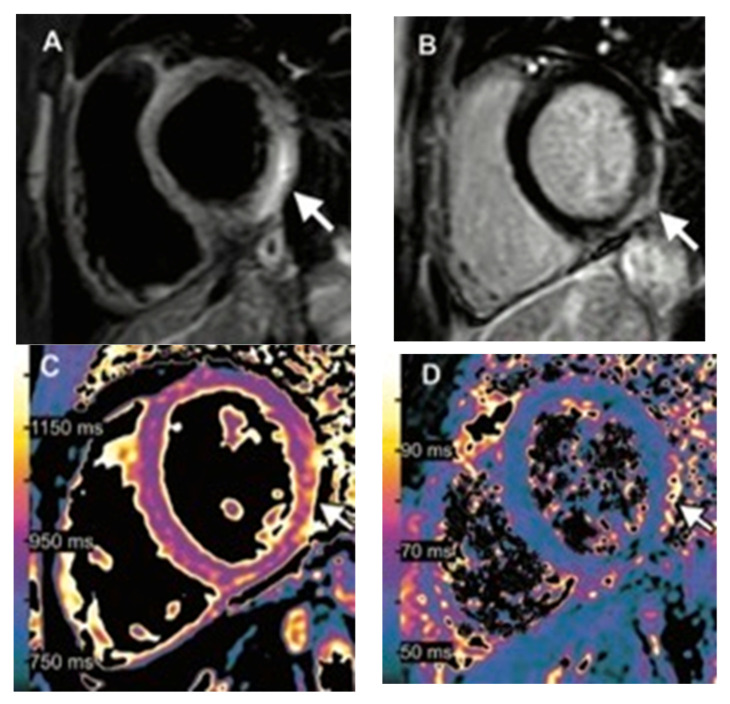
Images of a 15-year-old boy with myocarditis after COVID-19 vaccination. One day after receiving his second vaccination dose, he developed fever, myalgia, and intermittent tachycardia. (A) T2-weighted short inversion time inversion recovery MRI scans at 1.5 T in short-axis view show focal high-signal intensities (arrow) at the basal lateral and inferior wall, indicating myocardial edema. (B) Late gadolinium enhancement image in short-axis view shows corresponding linear subepicardial enhancement (arrow), indicating inflammatory myocardial necrosis. (C) T1 mapping and (D) T2 mapping in the short-axis view show elevated T1 and T2 at the mid-ventricular lateral and inferolateral wall (arrow in (C,D)), indicating acute myocardial injury (focal T1, 1165 ms; focal T2, 70 ms; institution-specific cut-off values for acute myocarditis: T1 global ≥ 1000 ms, T2 global ≥ 55.9 ms). Reprinted/adapted with permission from Ref. [8], 2021, RSNA.
